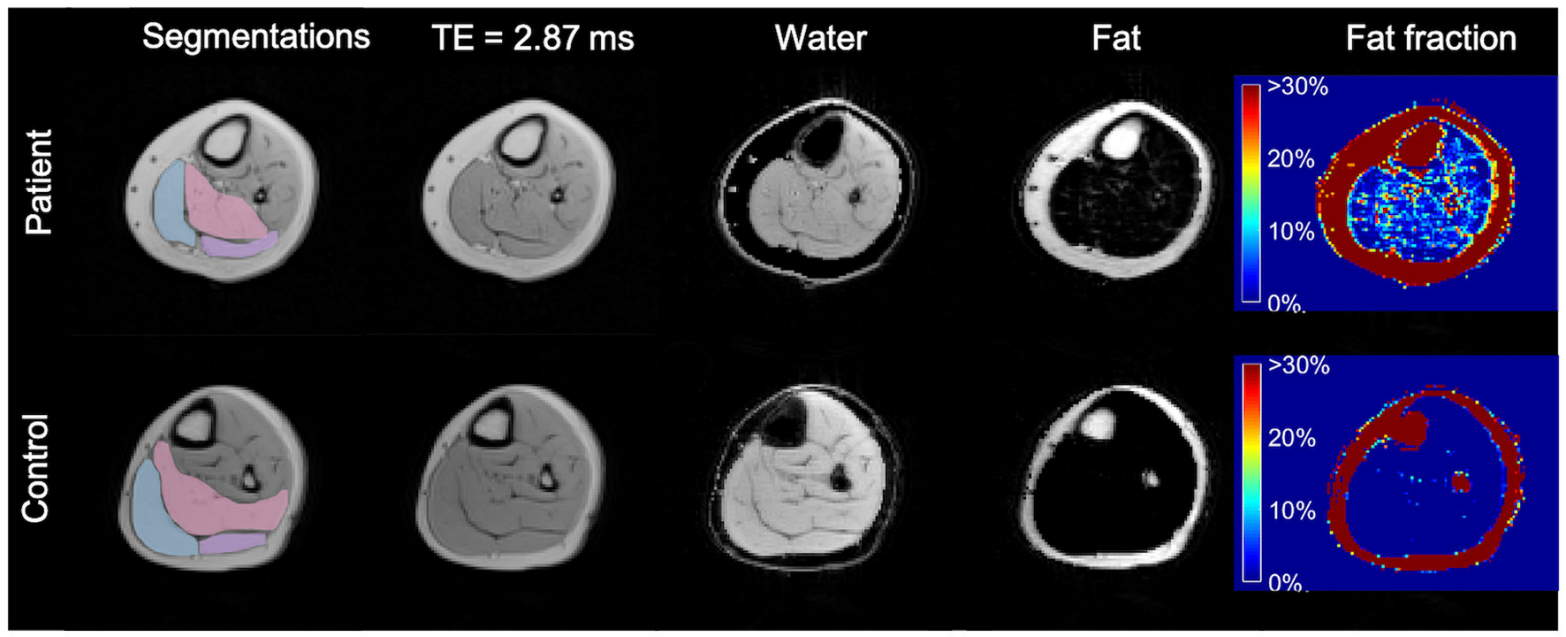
Axial MR images of the more affected calf of a patient (diparetic, boy, 11 years) and the dominant calf of healthy control (a typically developing 11 years boy with similar BMI). First column: segmented ROIs for soleus (pink), gastrocnemius medialis (light blue), and gastrocnemius lateralis (lilac); second column: 2nd echo image from the Dixon data set; third column: water-only image calculated from the Dixon dataset; fourth column: fat-only image calculated from the Dixon dataset; fifth column: fat fraction map ranging from 0 to 100% calculated from the Dixon dataset, showing a higher fat fraction in the CP patient. TE, Echo Time.
